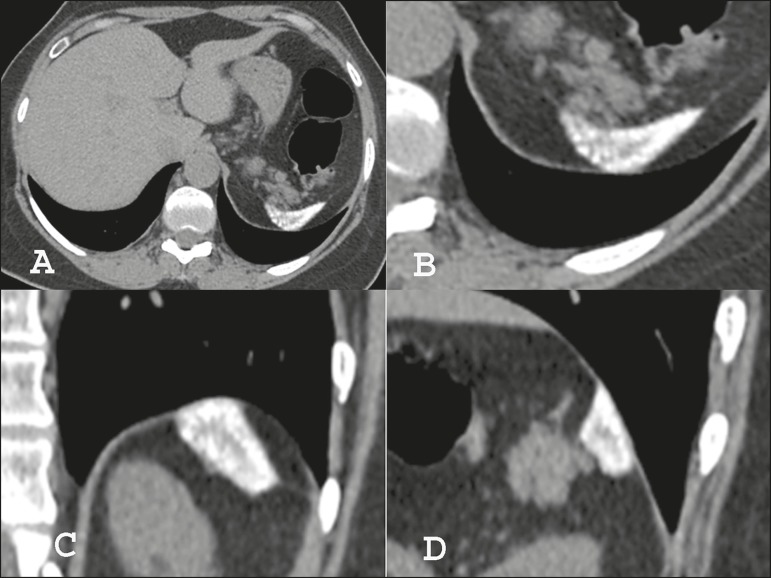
Computed tomography of the chest with mediastinal window settings
